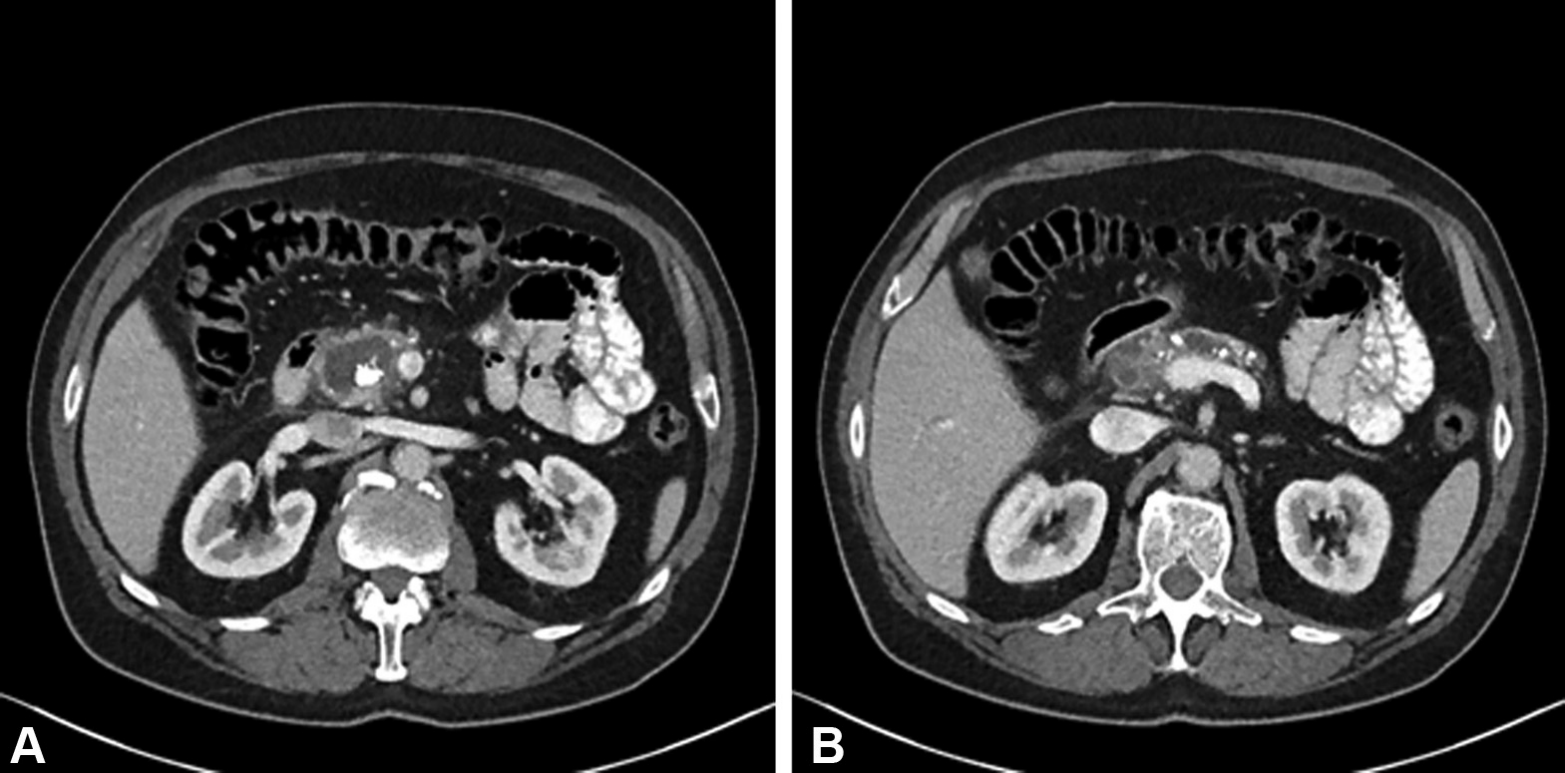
A andB: CT of the abdomen shows two obstructive pancreatic duct stones with upstream dilatation of the main pancreatic duct. Image A shows a pseudocyst in communication with the pancreatic duct.
